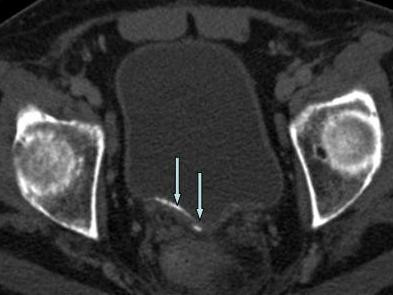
Axial pelvic CT scan with contrast. Note the linear calcific densities within the dependent portion of the urinary bladder (arrows).
